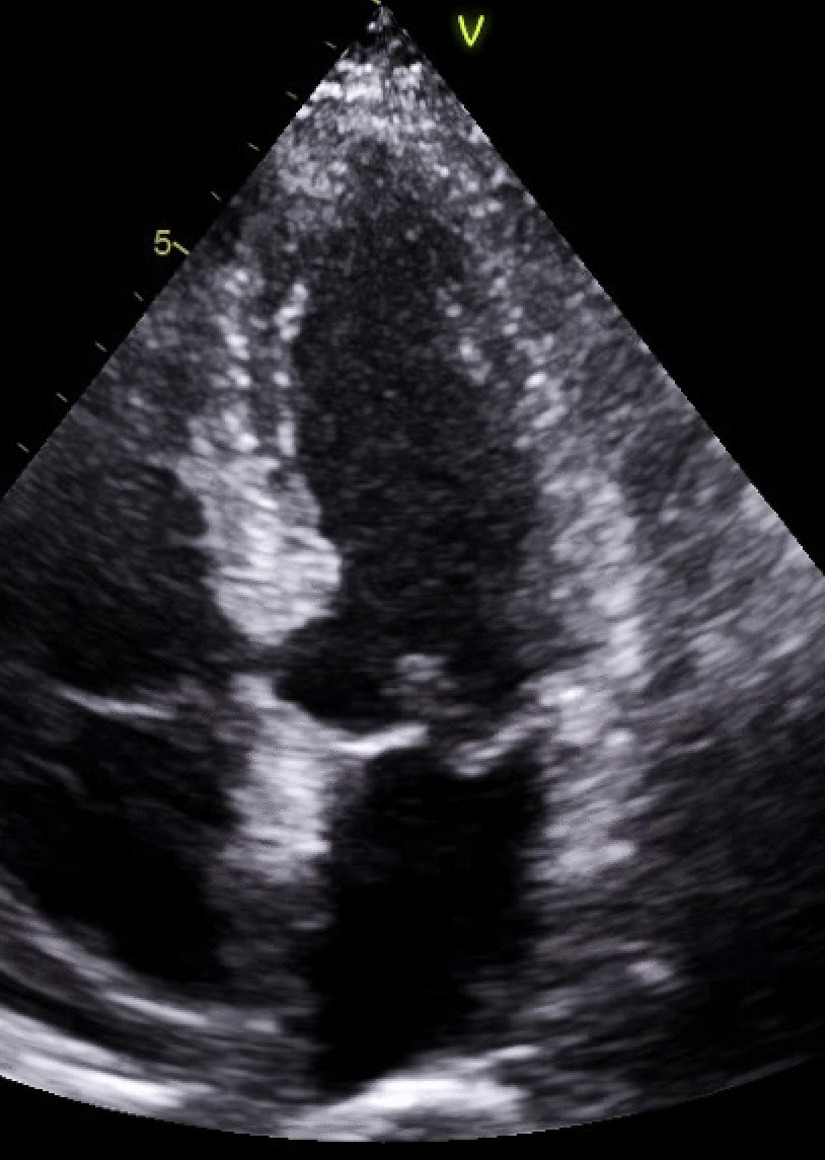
Case 8: Figure 1. Transthoracic echocardiogram (TTE) four chamber at end diastole. Mild asymmetric LV hypertrophy
